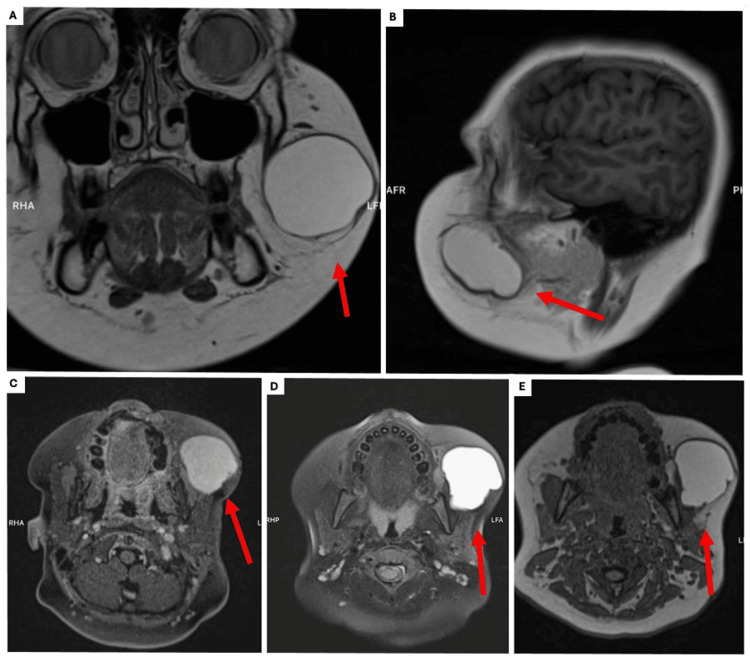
Magnetic Resonance Imaging (A) An oval, well-defined lesion with a thin wall was found in the left maxillary soft tissue on the coronal view on T1. (B) T1 on the sagittal view. (C) T1 on axial view. (D) The same lesion was hyperintense also on T2. (E) No enhancement with contrast and no infiltration of the surrounding tissue.
